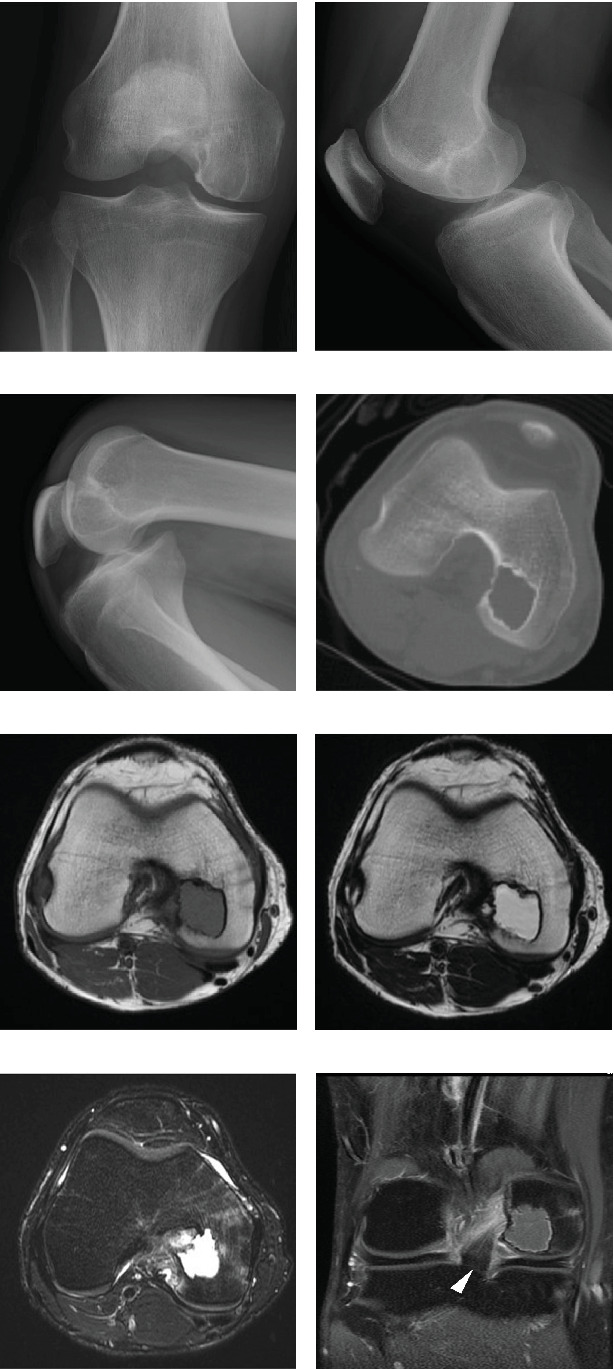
Frontal (a) and lateral (b) views of radiographs showing a well-demarcated radiolucent lesion at the intercondylar side of the medial condyle. (c) Maximal flexion of the knee advanced the lesion anteriorly, providing good view in our surgical field. (d) Axial view of the computed tomographic image showing an epiphyseal lesion with a sclerotic border. An intercondylar approach provided better access to the lesion than a conventional extra-articular approach. Axial view of the magnetic resonance image showing an epiphyseal lesion with low signal intensity on the T1-weighted image (e) and high signal intensity on the T2-weighted image (f). (g) The surrounding bone marrow showed high intensity with the fat-suppressed T2-weighted image. (h) Coronal view of the contrast-enhanced fat-suppressed T1-weighted image showing the tumor at the medial condyle and near the insertion site of the posterior cruciate ligament at the intercondylar fossa.
